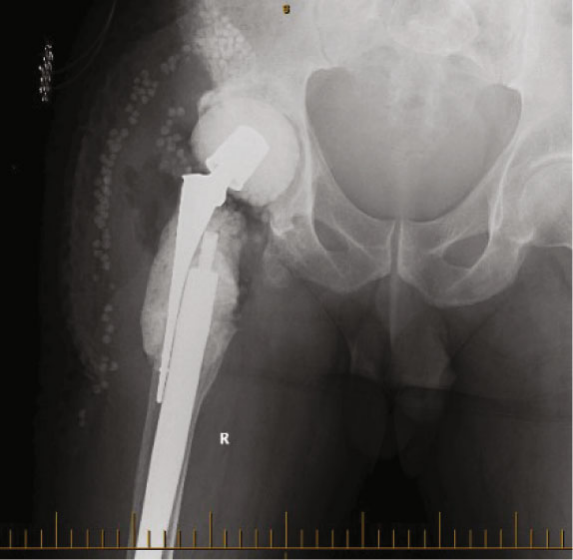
Pelvic X-ray of the patient post first-stage revision with cement spacer and retained stem 2016.
